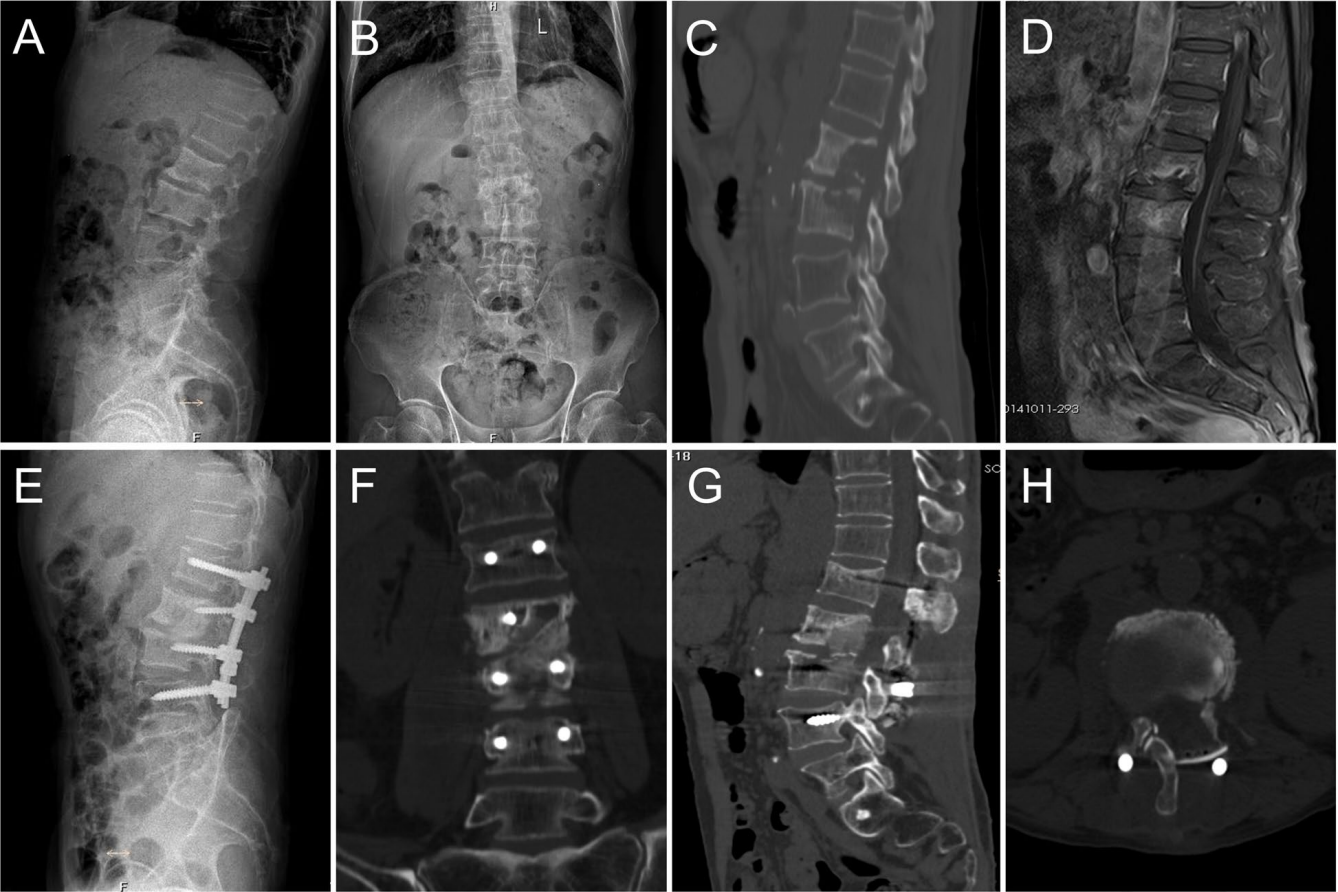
An aged male with destructive L2-3 was treated by posterior unilateral limited laminectomy debridement and bone grafting fusion combined with internal fixation. Figure 5 A, B, C, and D are preoperative images showing lesion and destructive vertebra at the L2 and L3 vertebrae. Two years after the operation, the X-ray (E) and CT (F, G, H) showed a solid bone fusion
